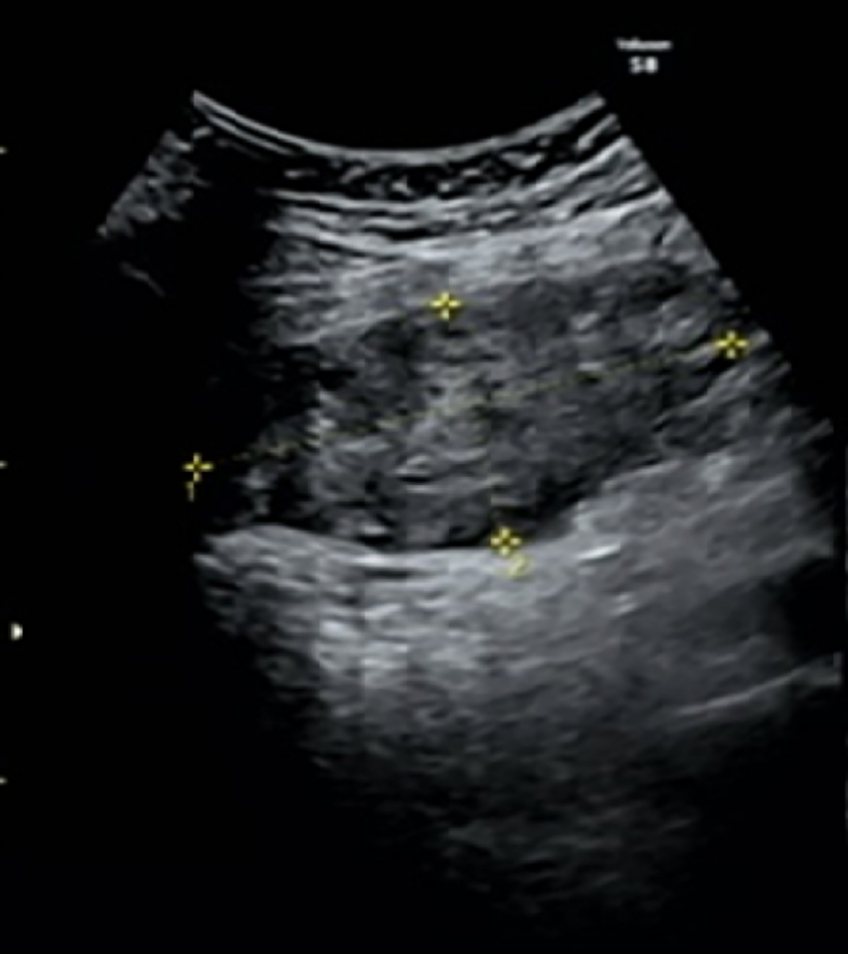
Transabdominal ultrasonography revealed a non-homogeneous low-echoic mass in the right adnexal region.
